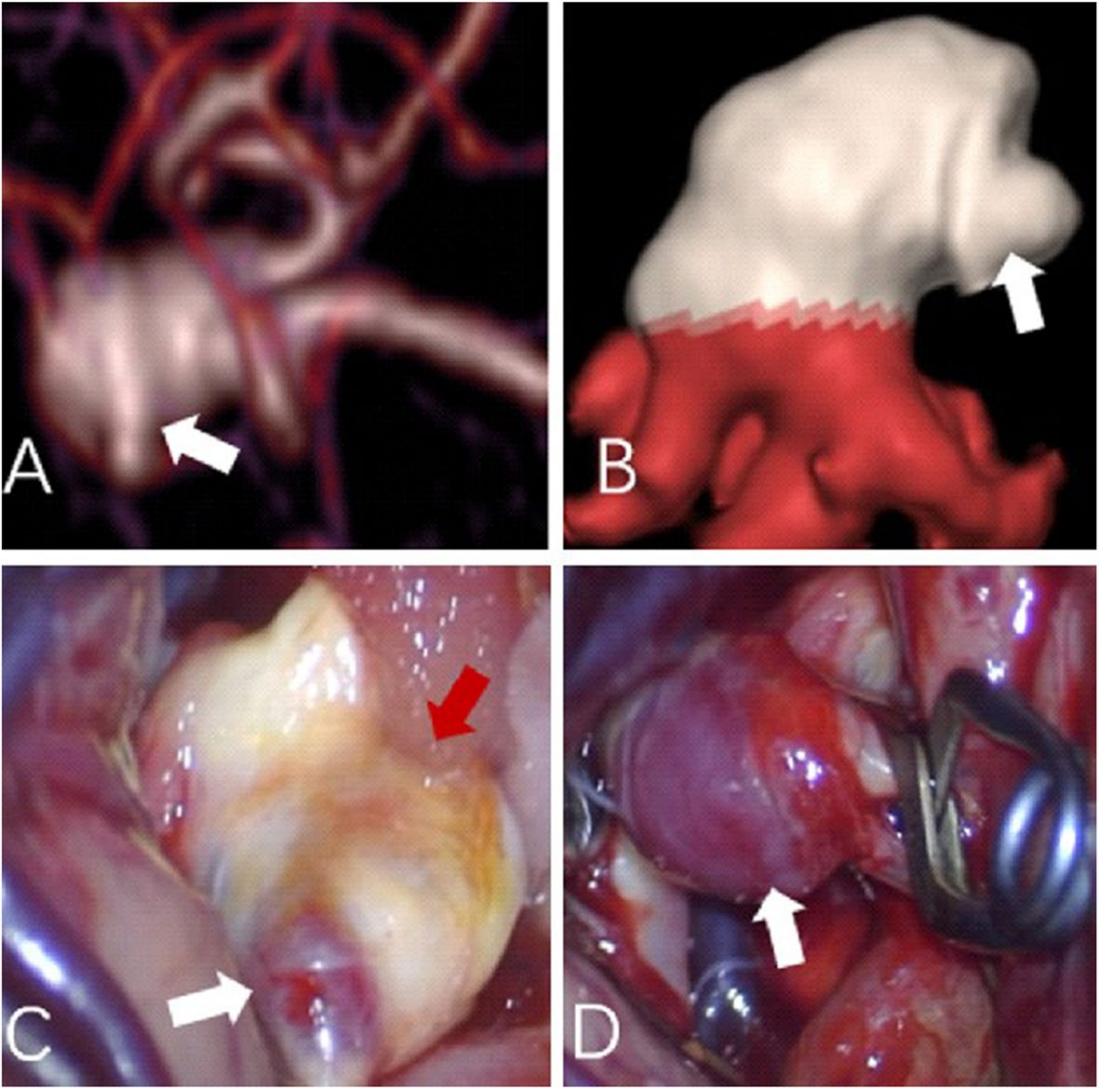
A-B CTA showed a left MCA-M1 aneurysm (10.8 mm x 5.7 mm), and an obvious pulsation was observed by 4D CTA (as indicated by the white arrow). C-D Photographs obtained during surgery, small vesicles shaped convex and calcified plaque were seen intra-operation (as indicated by the white and red arrow, respectively), an intra-operational finding confirmed that the abnormal pulsation site is an at-risk aneurysmal wall
